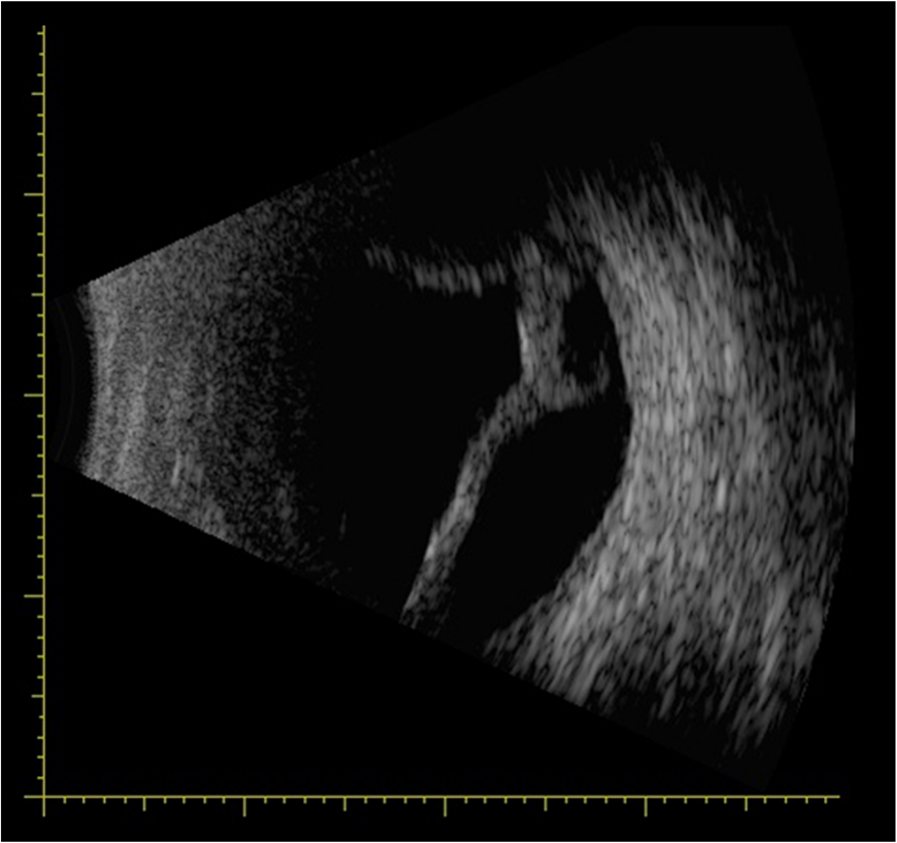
Preoperative B-scan ultrasonography showing 360 °choroidal detachment
